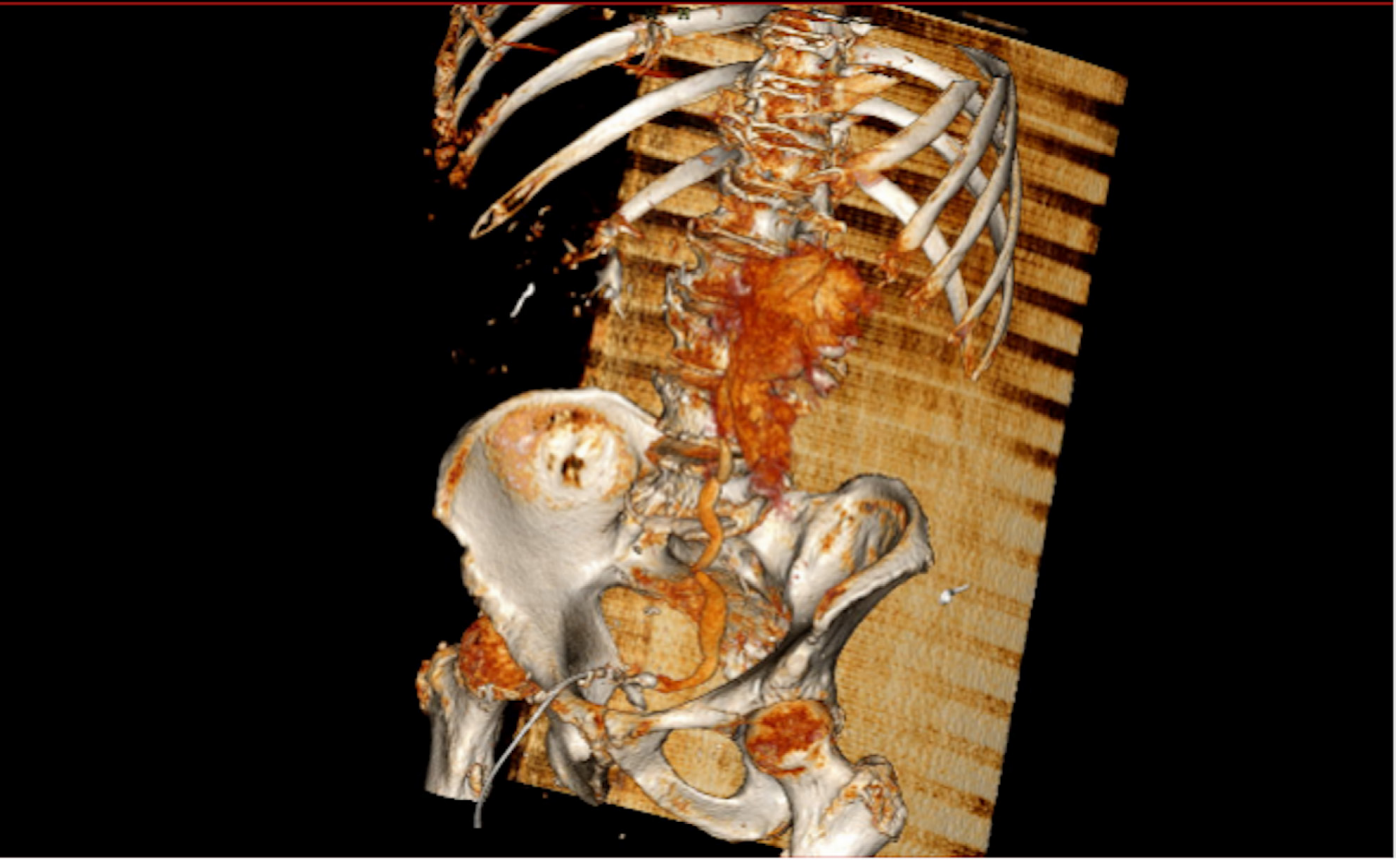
CT Urogram—VR reconstruction; delayed phase image demonstrating contrast extravasation from the left renal calyx and hold up of contrast in the left excretory system due to stone in distal ureter
